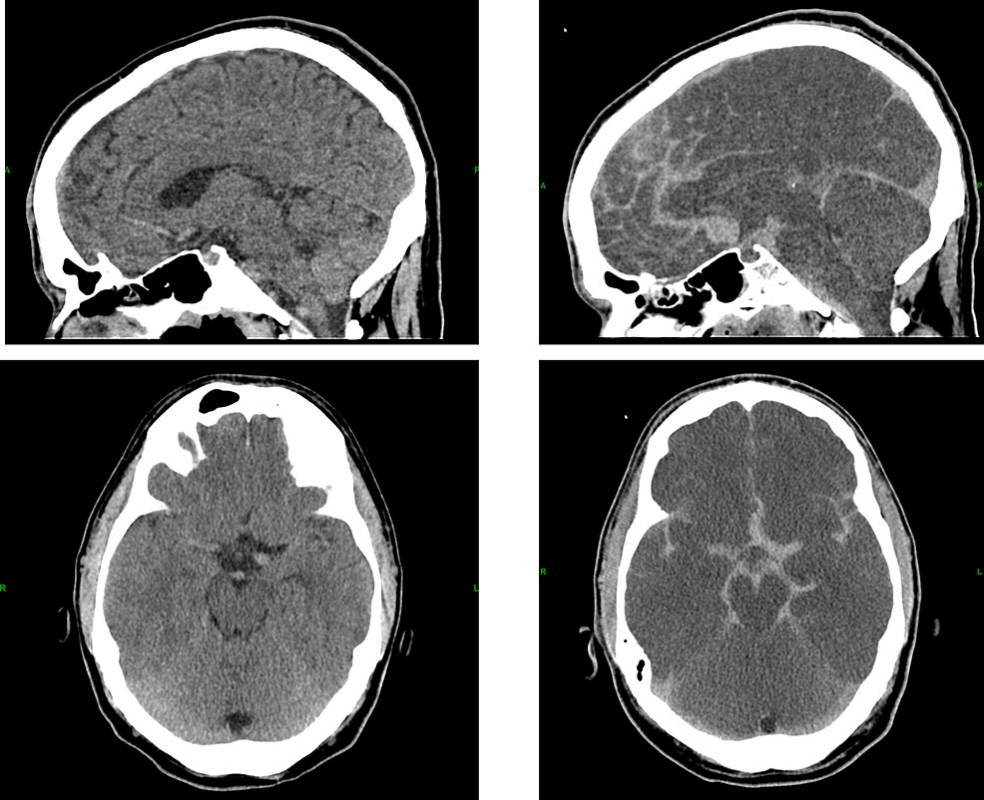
(Right) Shows extensive intracranial subarachnoid hemorrhage visualized during hospitalization, compared to (left) of CT head one year prior CT, computed tomography
